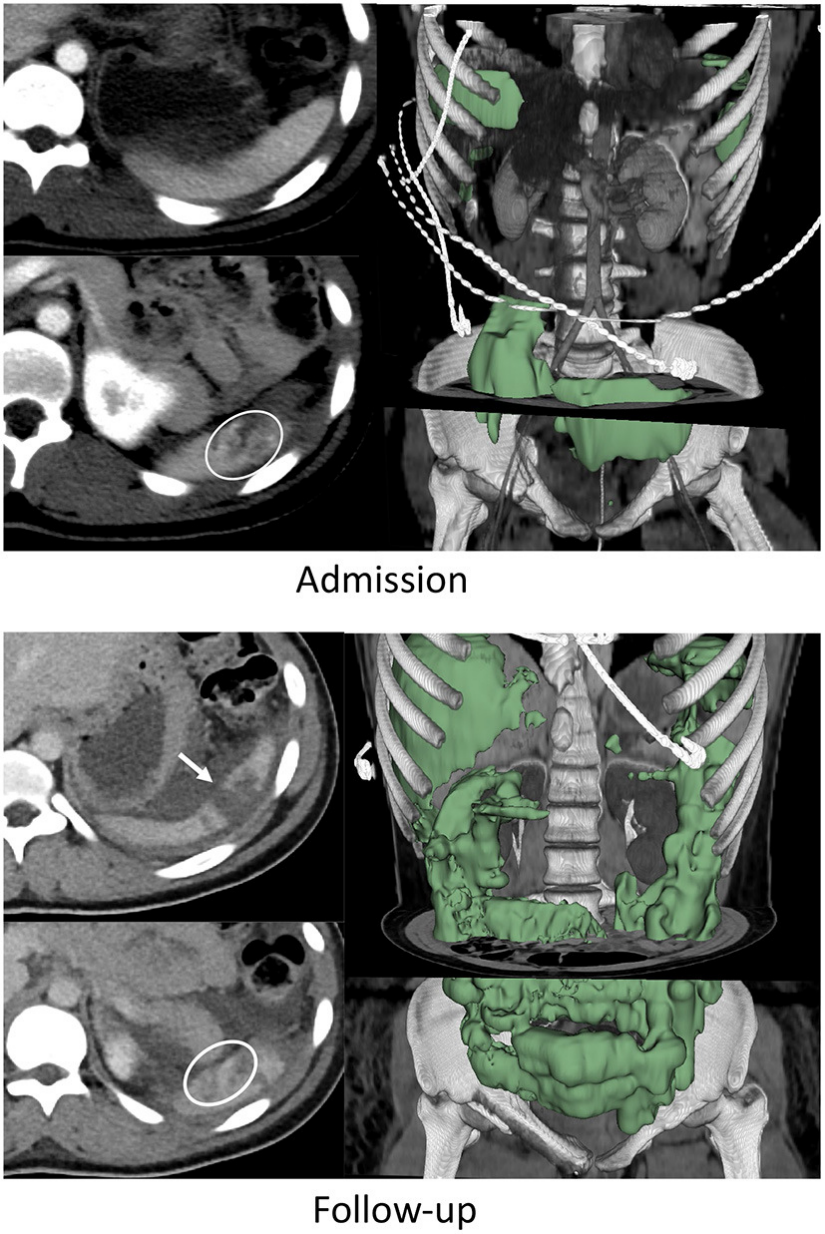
Nineteen years-old female with BSI who failed AE and underwent splenectomy. On admission CT, the patient had a grade IV BSI with several pseudoaneurysms in the inferior pole of the spleen (circle on bottom left admission CT image), and 253 mL of hemoperitoneum. This increased to 1,415 mL on follow-up CT performed 49 h later (green label mask, right image). Pseudoaneurysms were no longer visualized but the laceration had expanded to include the upper pole (arrow on top left follow up image).
